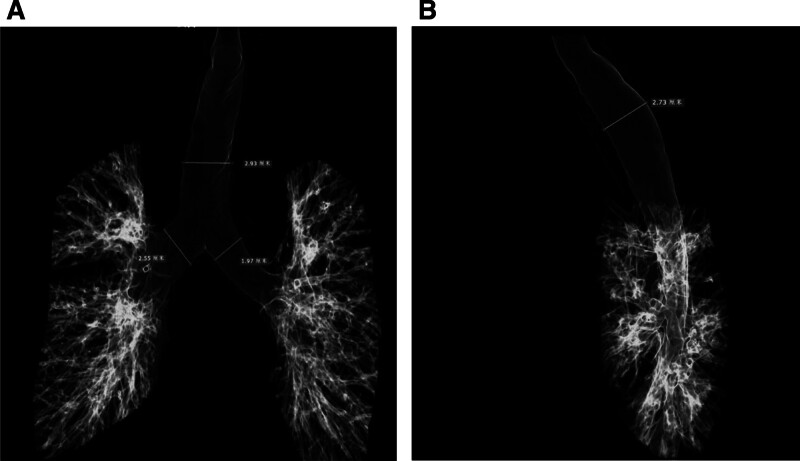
(A) Three-dimensional reconstruction using the CT airway transparency technique demonstrates severe dilatation of the trachea and bronchi. The maximal coronal diameter of the trachea measured 29.3 mm, while the left and right main bronchi exhibited coronal diameters of 19.7 and 25.5 mm, respectively. (B) The maximal sagittal diameter of the trachea reached 27.3 mm. CT = computed tomography.
